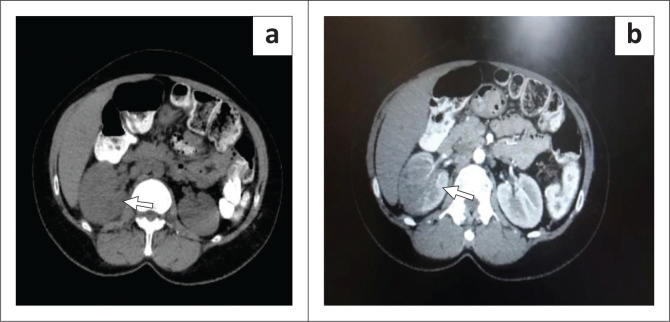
Radiological imaging: (a) Computed tomography scan show iso-dense heterogeneous renal mass (arrow); (b) Magnetic resonance imaging show iso-intense right renal mass with solid and cystic areas (arrow).
